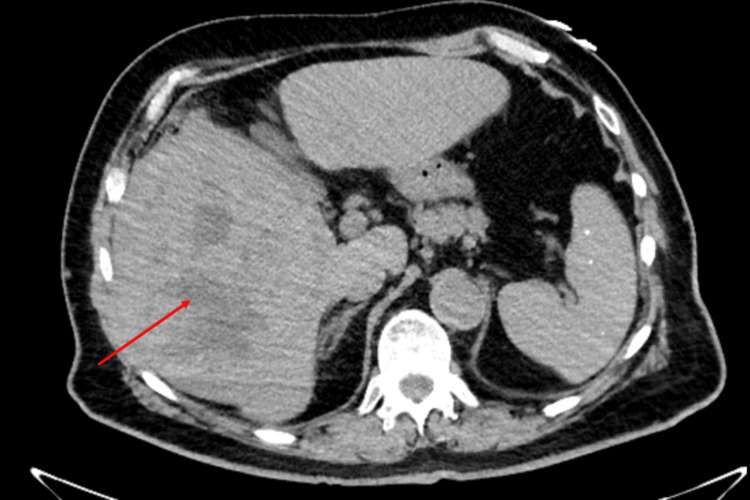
Axial CT of the abdomen showing a large right hepatic lobe mass (arrow) consistent with HCC CT: computed tomography, HCC: hepatocellular carcinoma
